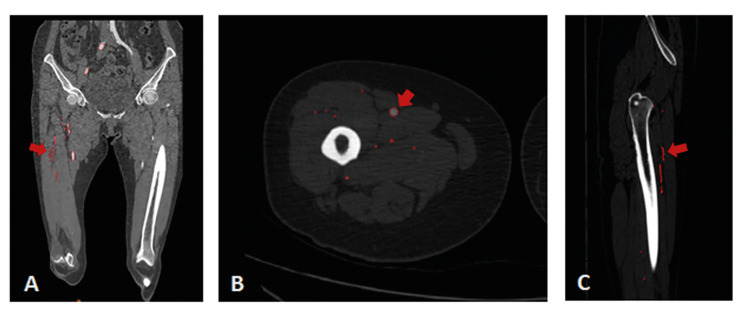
The red arrows in CTA imaging data refer to the perforator vessel of ALT flap located preoperatively (A. coronal plane; B. horizontal plane; C. sagittal plane).
